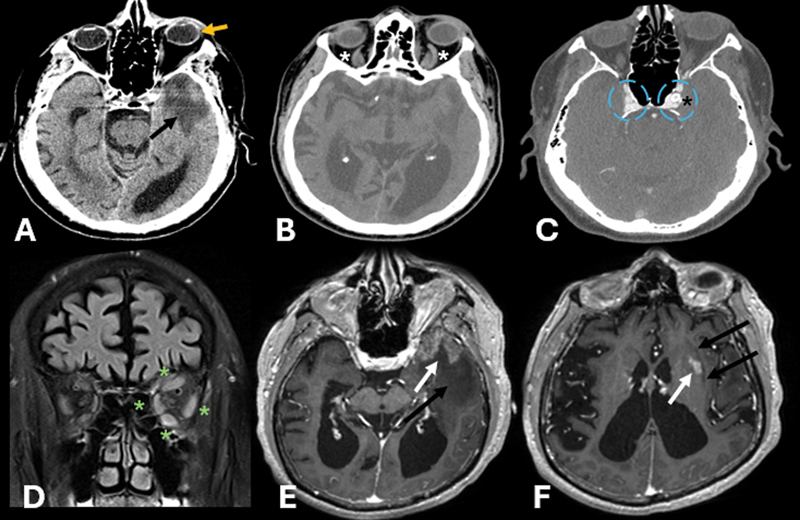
Axial non-contrast computed tomography (CT) scan (
